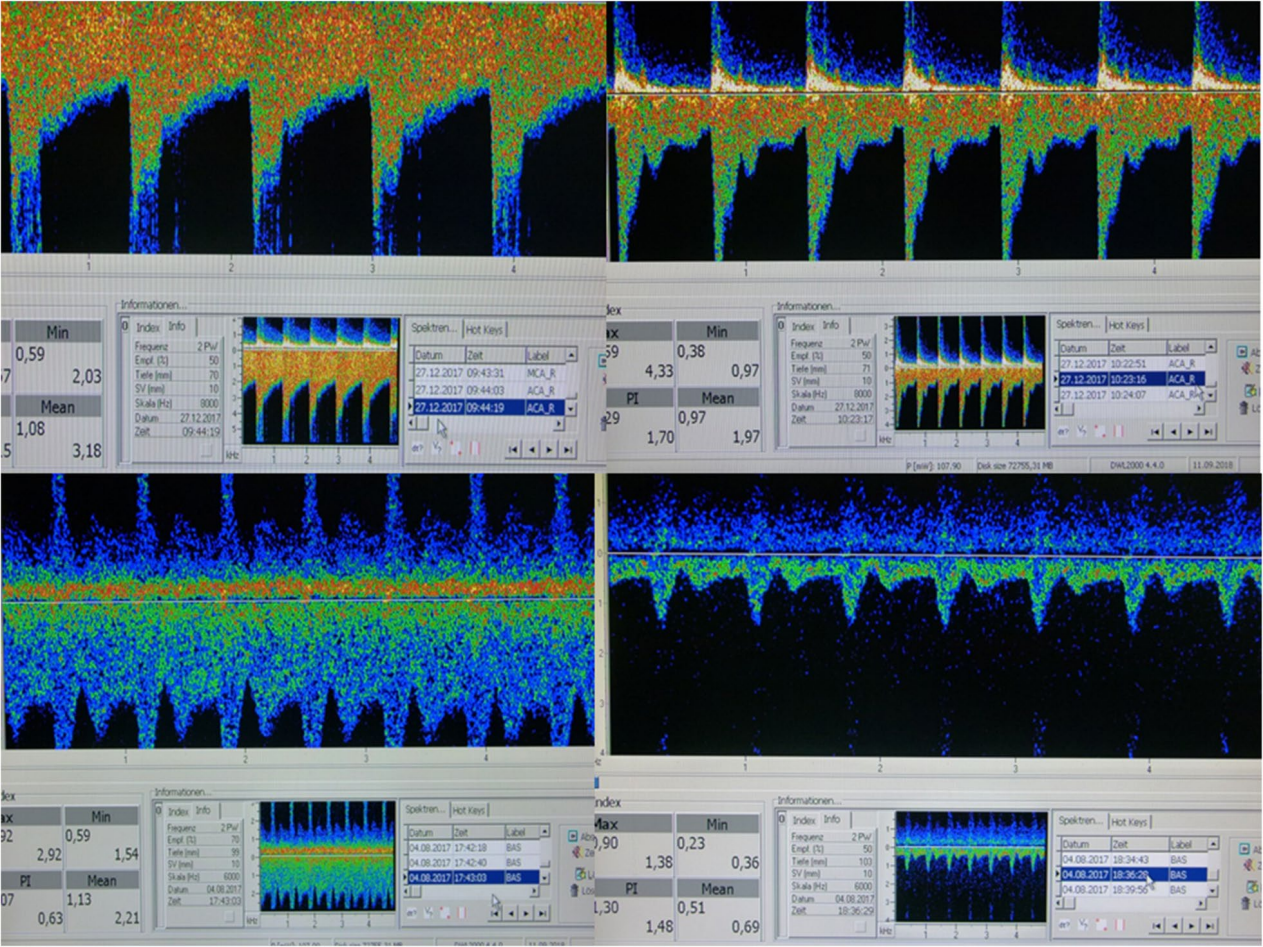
Transcranial Doppler ultrasound before and after sodium nitroprusside bolus. This figure depicts examples of transcranial Doppler sonography changes prompting an intraventricular administration of SNP bolus and its results. Upper panel: 48yo male, day 3 after subarachnoid hemorrhage, Glasgow Coma Scale-3, Hunt and Hess V, intracranial pressure 8 mmHg, mean arterial pressure 76 mmHg, Molsidomine 6 mg/h, no vasopressors. Left panel, 9:44 am: the right anterior cerebral artery prior to sodium nitroprusside; mean flow 318 cm/s. Right panel, 10:24 am: after 2 × 5 mg sodium nitroprusside, mean flow 197 cm/s, the clear shape of the signal. After the second bolus of sodium nitroprusside, 170 mg Urapidil. Lower panel: 56yo male, day 6 after subarachnoid hemorrhage, Glasgow Coma Scale-5, Hunt and Hess IV, intracranial pressure 17 mmHg, mean arterial pressure 97 mmHg, Molsidomine 16 mg/h, no vasopressors. Left panel, 5:43 pm: the basilar artery prior to sodium nitroprusside, mean flow 221 cm/s. Right panel, 6:36 pm: after 5 mg sodium nitroprusside, mean flow 69 cm/s. After sodium nitroprusside, 180 mg Urapidil
